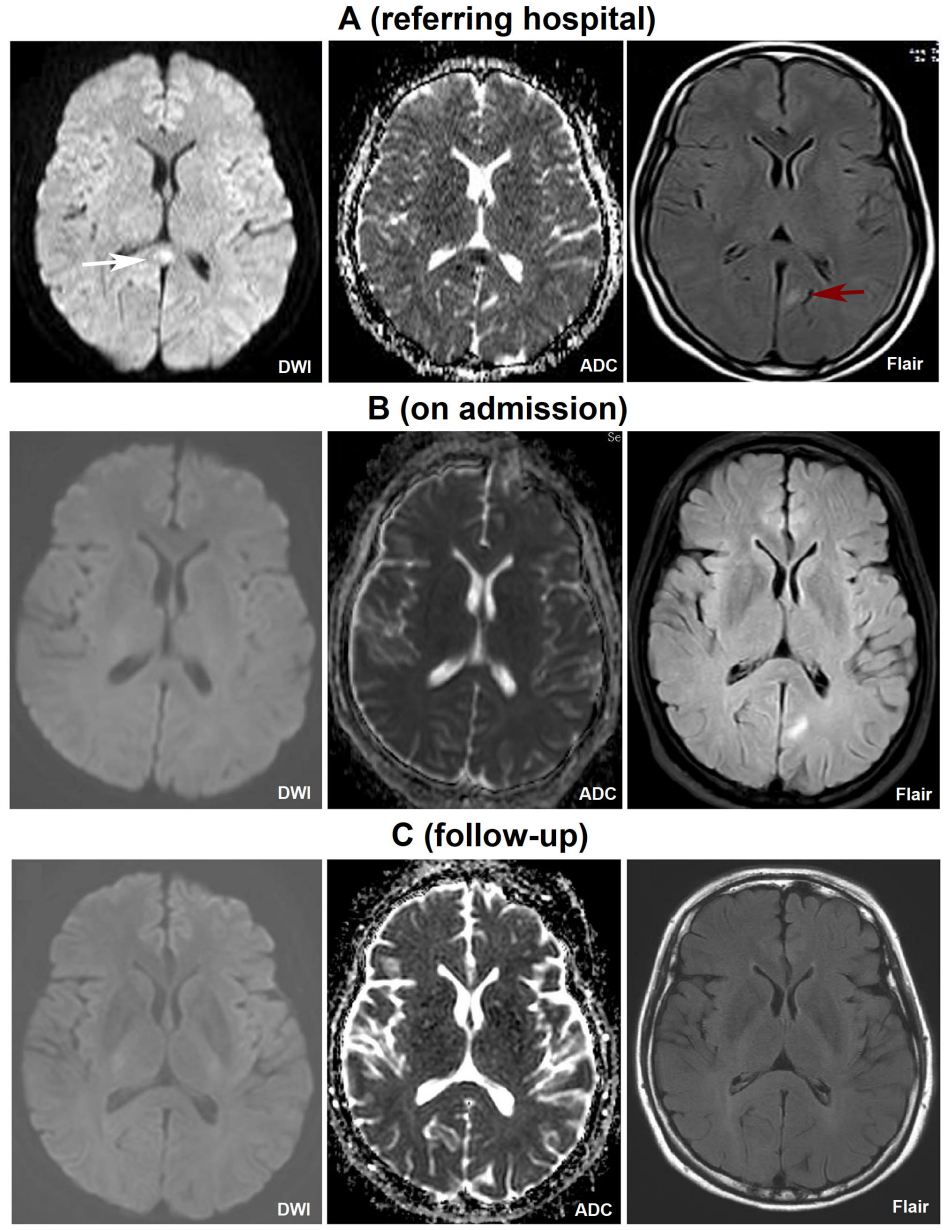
Brain MRI images at different time-points. Images taken from the referring hospital (A), on admission (B) and 3-month follow-up (C). The MRI signals showing cytotoxic edema in the SCC (white arrow), characterized by hyperintensity in the DWI sequence and hypointensity in the ADC sequence, resolved rapidly. In contrast, the signal indicating acute demyelination in the SCC and left occipital lobe (red arrow), identified by hyperintensity in the Flair sequence, persisted for a longer period. MRI, magnetic resonance imaging; SCC, the splenium of the corpus callosum.
